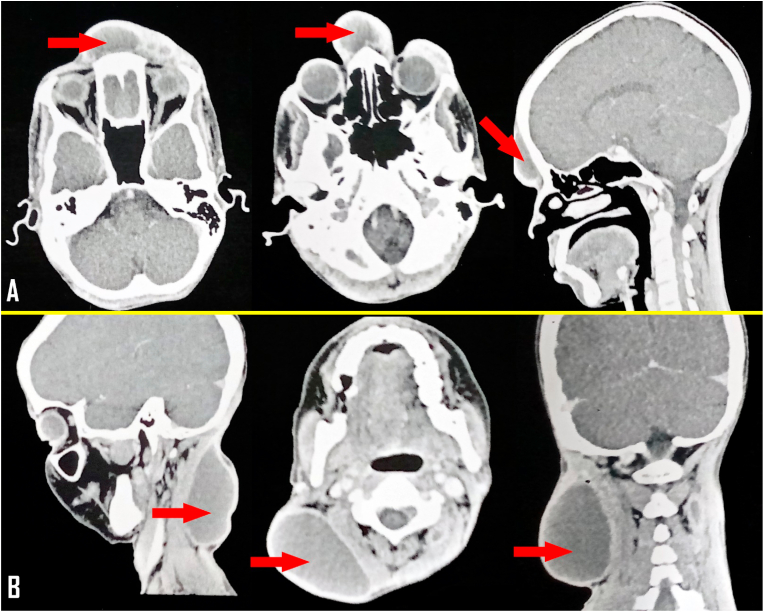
Cranio-cervico-facial CT scan showing a fronto-glabellar and right posteriour cervical subcutanous collections.
